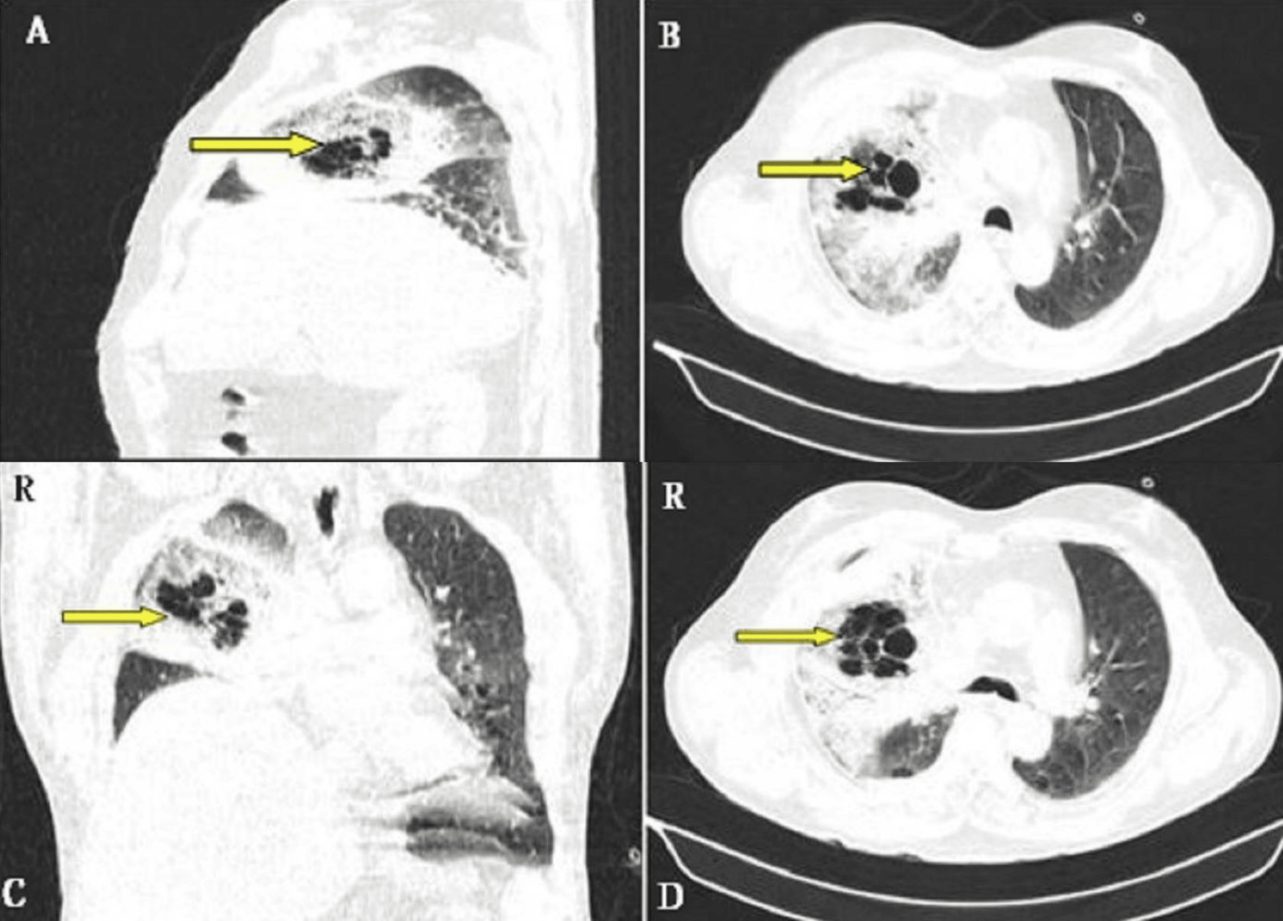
High-resolution computed tomography of the thorax showing multiple cystic cavitations with surrounding consolidation in a nest pattern described as a bird's nest sign (yellow arrows).
